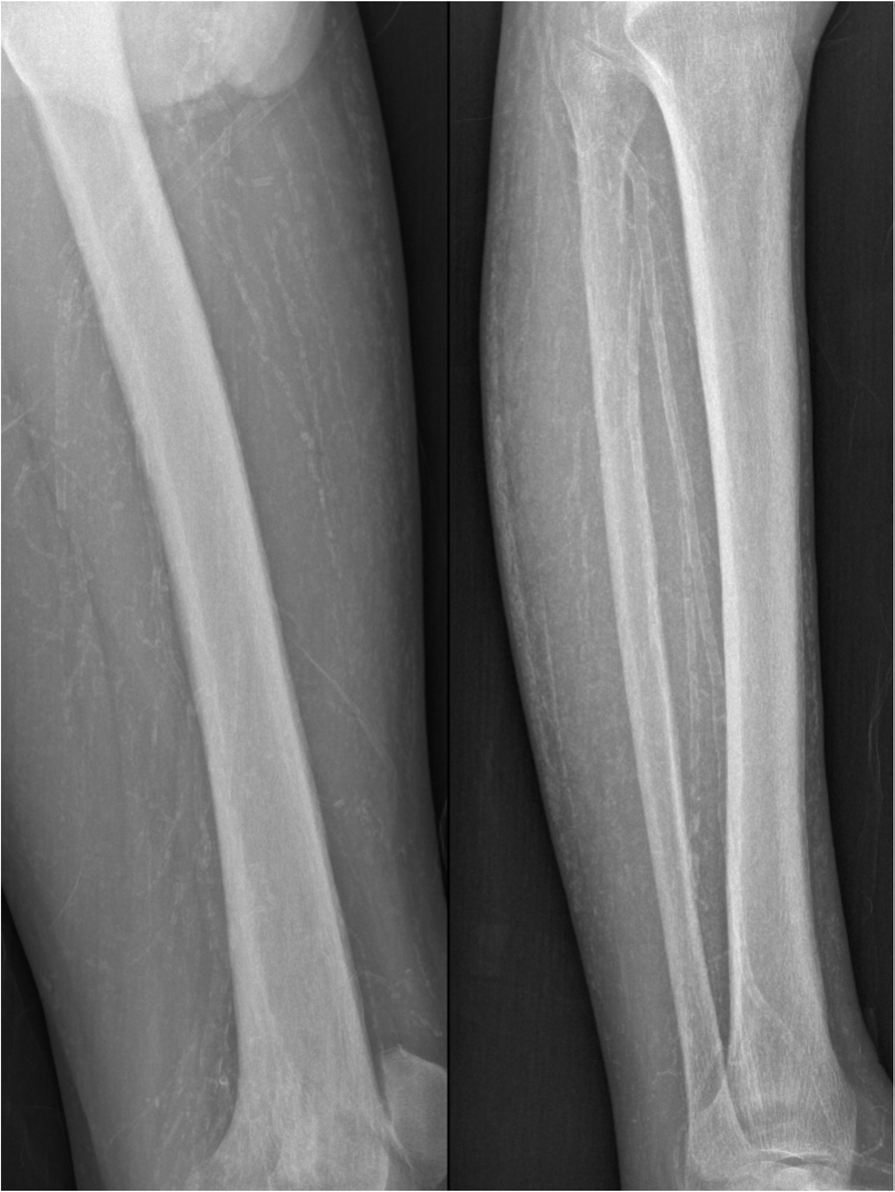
Plain radiographs of the left thigh and calf show that small-vessel calcification and a net-like pattern of calcification can be observed beyond the locus of ulcer formation
